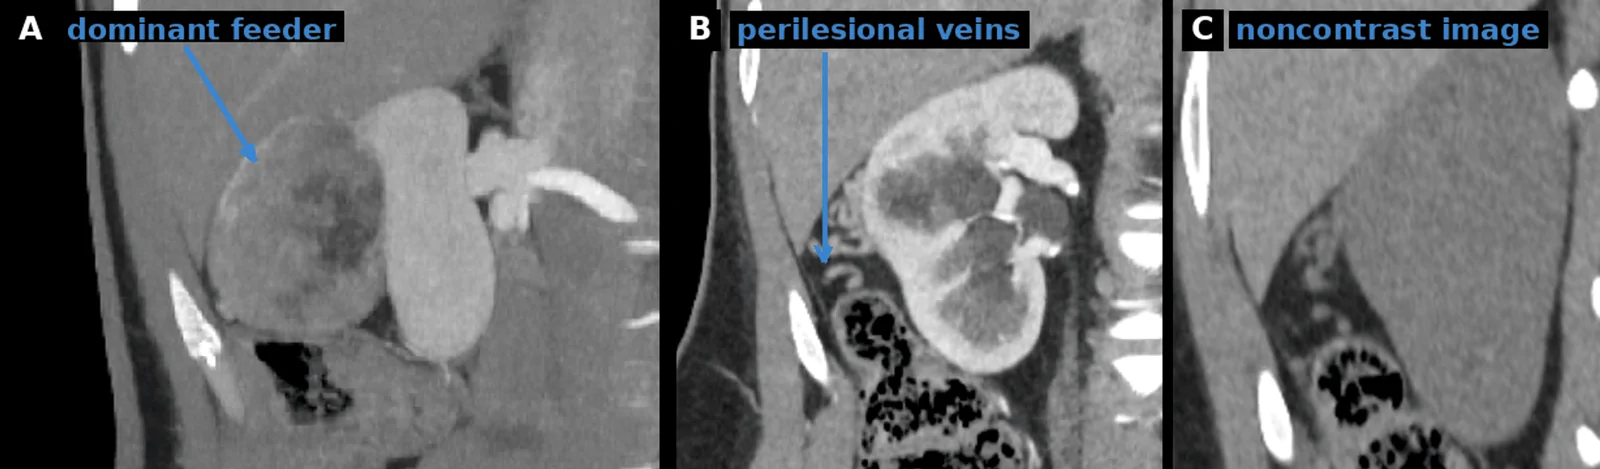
Preprocedural CTA A multiplanar reformatted image demonstrates the dominant arterial feeder (A). Arterial-phase imaging demonstrates early opacification of enlarged perilesional veins, raising suspicion of intratumoral arteriovenous communication (B); however, a discrete arteriovenous fistula or high-flow shunt was not directly demonstrated. The corresponding noncontrast image is provided for comparison (C). Blue arrows identify the arterial feeder and perilesional veins. CTA: computed tomography angiography; AV: arteriovenous.
